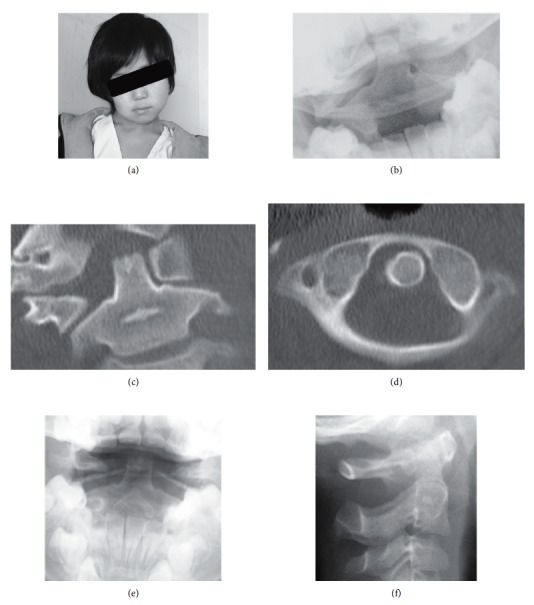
Patient showing torticollis before treatment, with head tilting, neck rotation, and a characteristic “cock-robin” position (a). Simple cervical open mouth view radiograph showed lateral tilting of the cervical spine and rotated atlas on the axis (b). Coronal (c), axial (d), and 3D (e). CT images showed lateral tilting of C1 and anterior rotated atlas on the axis. Plain radiograph after closed reduction under general anesthesia (e, f).
